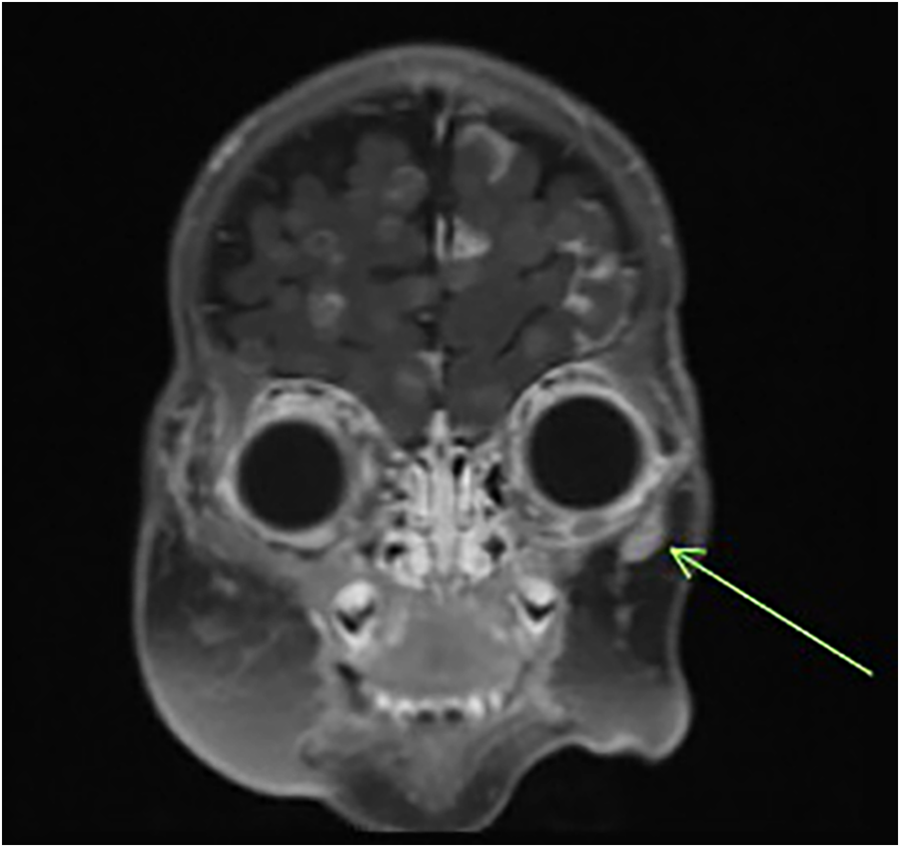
Follow-up MRI of the brain/orbit demonstrating diffuse intracranial metastatic spread and enlargement of facial lesions.
